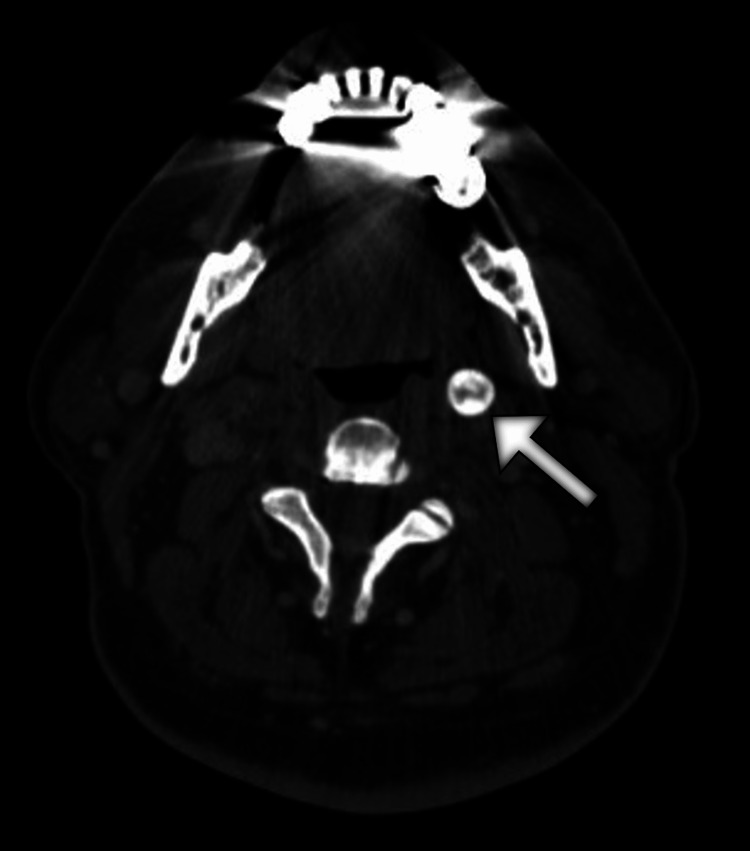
CT image of the neck shows a markedly thickened and prolonged left styloid process (arrow). CT: computed tomography
